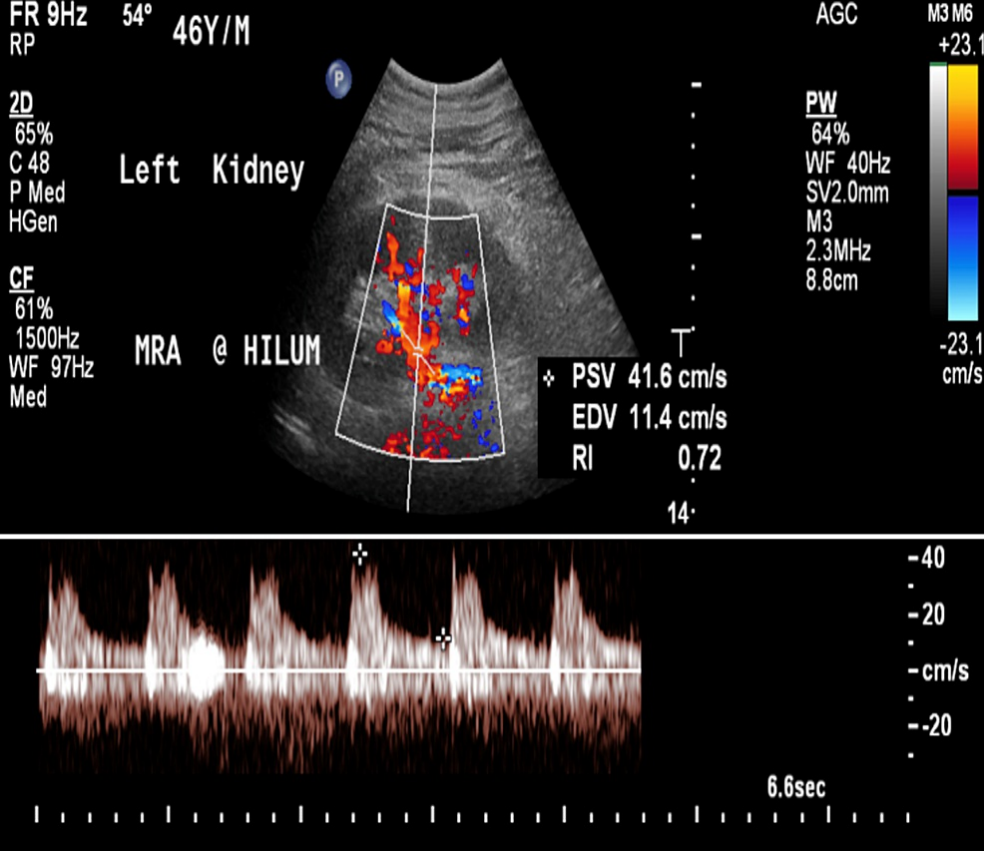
Ultrasound image with power Doppler showing normal flow in the renal artery.
